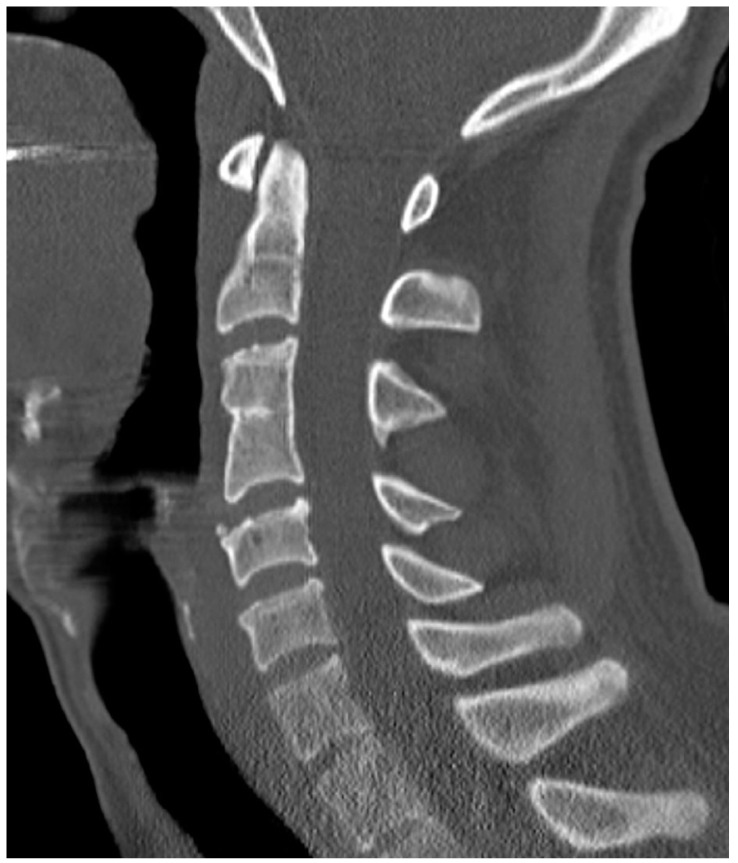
Sagittal CT reconstruction; 28 y/o female; congenital block vertebra C3/4 with degenerative changes in segments C4/5 and C5/6 representing a combination of ASDI and natural degenerative changes.
